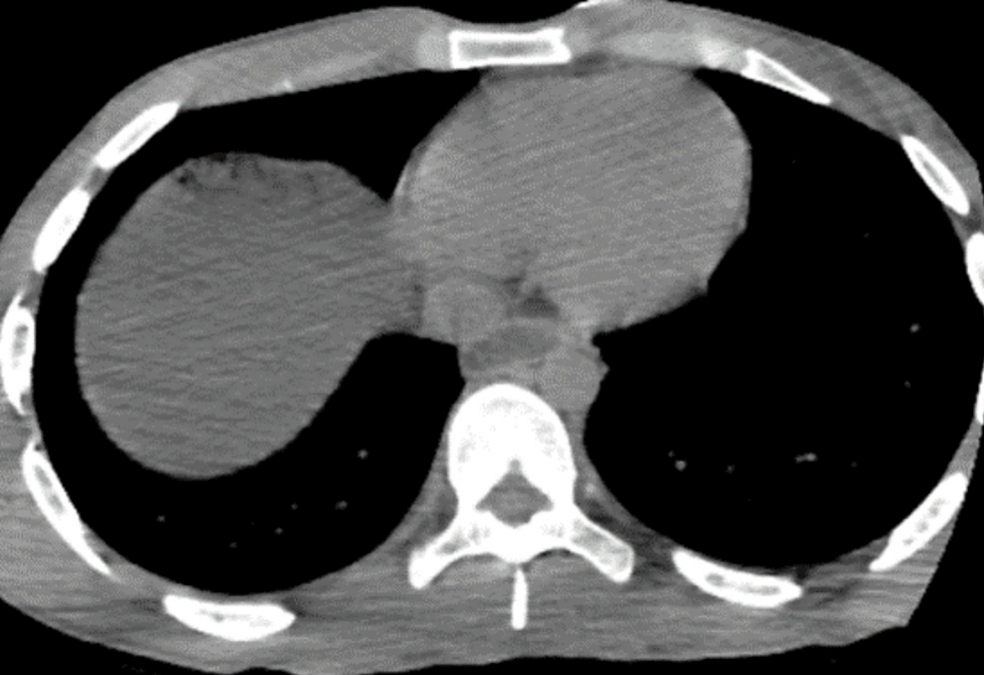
Axial noncontrast-enhanced CT of the thorax on day 1 showing no abnormalities.
